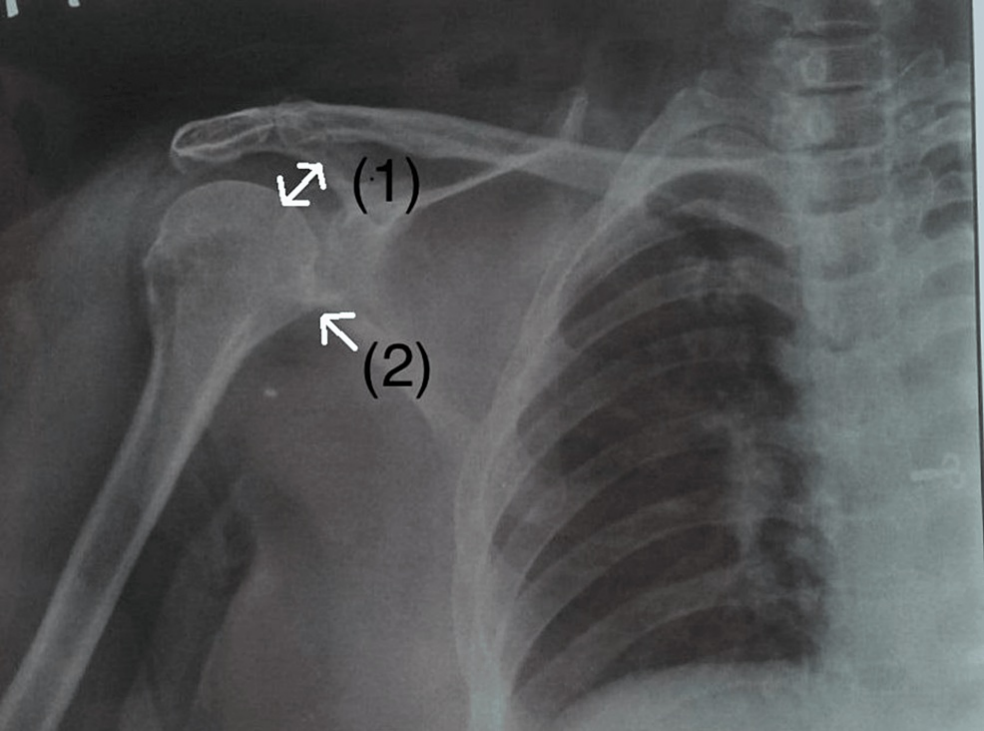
X-ray of the patient (i) Slight degenerative changes and (ii) slight decrease in the joint space which causes hypomobility.
